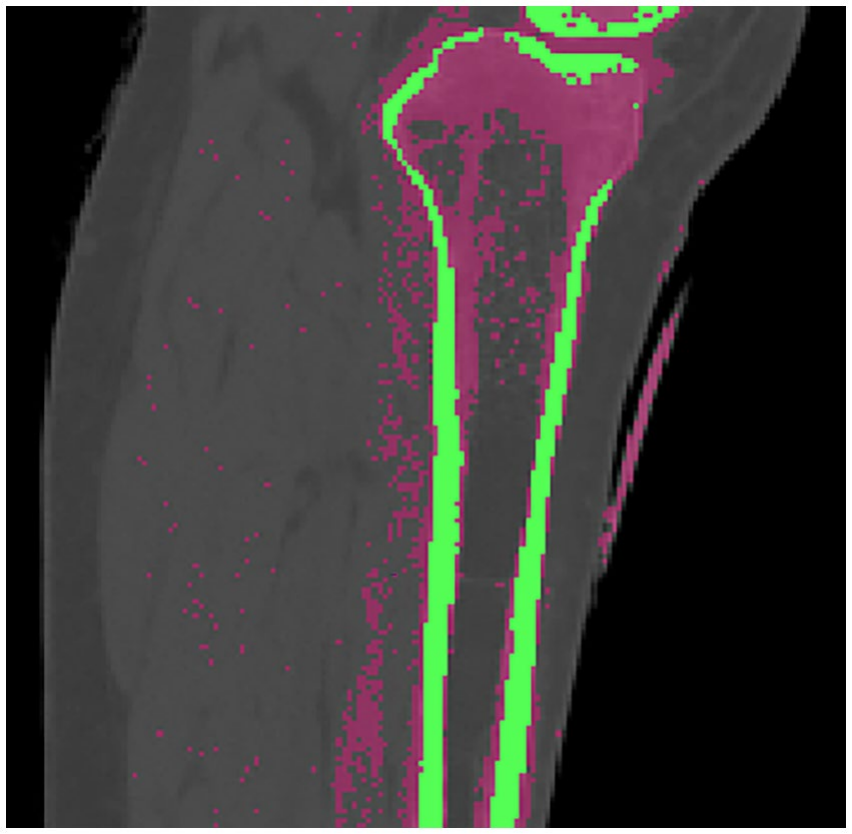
Cortical and cancellous bone were distinguished by different threshold values from the CT images, and the green part was cortical bone and the pink part was cancellous bone.
